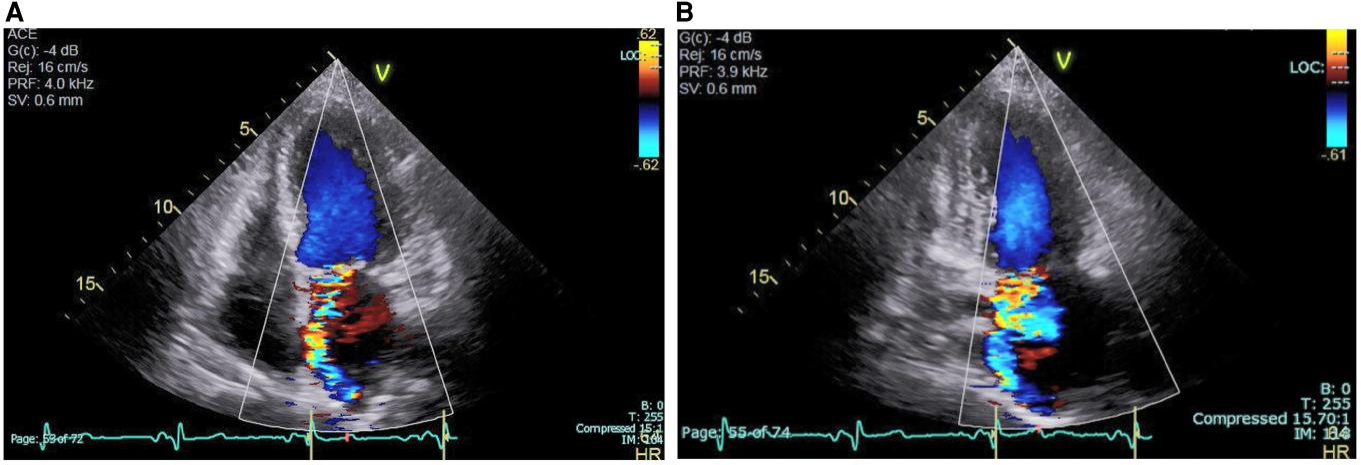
Transthoracic echocardiography. Colour Doppler imaging showed eccentric mitral regurgitation in (*A*) apical four-chamber and (*B*) two-chamber view.
